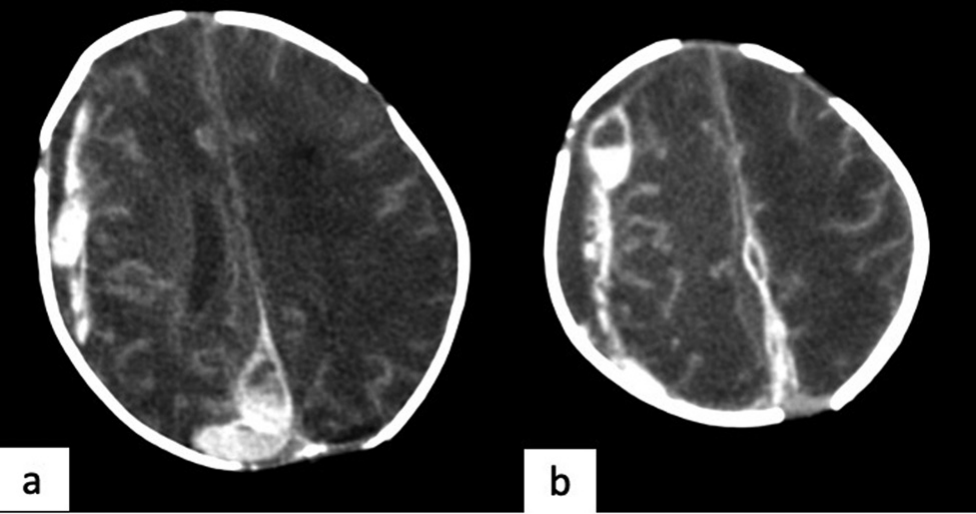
Evolutive hemorrhagic ischemic infarction. Axial computed tomography (CT) contrast images show extra-axial collection overlying (
